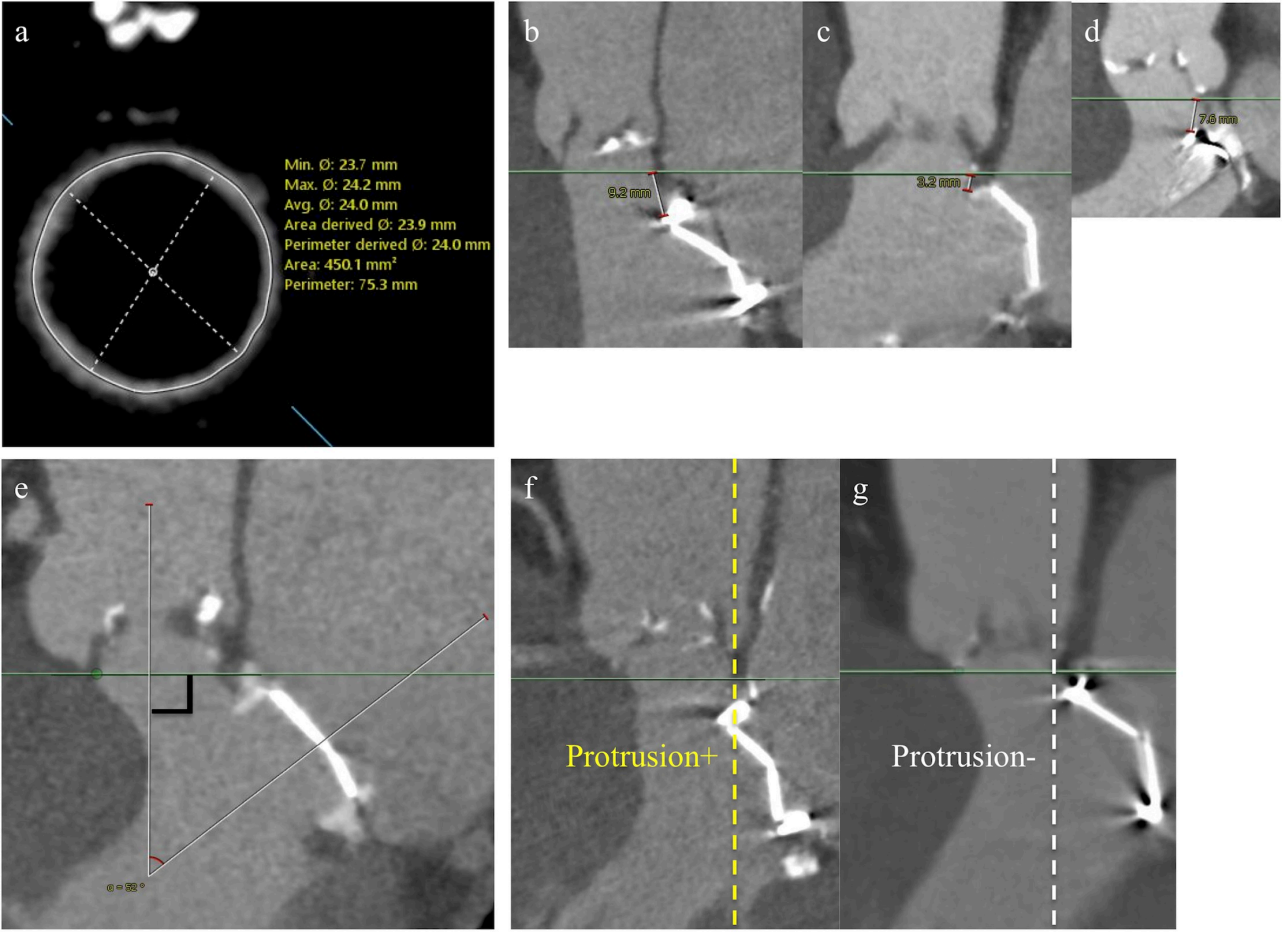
Preprocedural MDCT measurement. (a) Size of the mitral prosthesis housing. (b and c) The distance between the aortic annulus and mitral prosthesis housing. (d) The distance between the aortic annulus and the stem of the mitral bioprosthesis. (e) The angle between the mitral prosthesis and LVOT. (f and g) Assessment of mitral prosthesis protrusion to the LVOT. LVOT = left ventricular outflow tract; MDCT = multidetector computed tomography.
